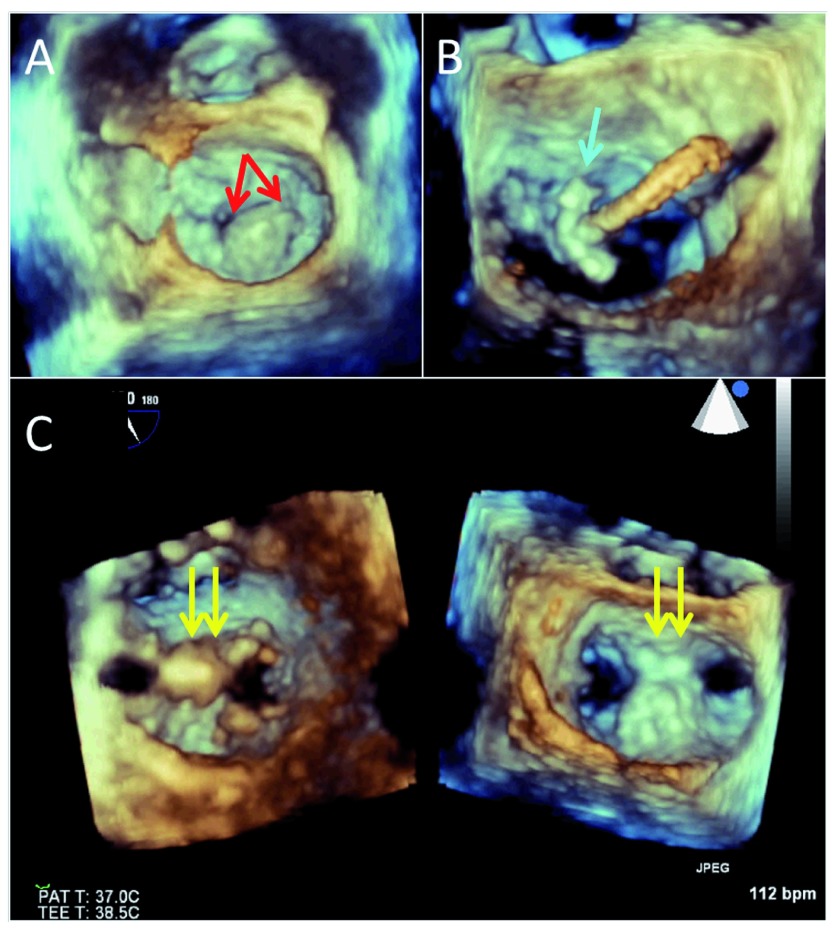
Three-dimensional echocardiography during a transcatheter mitral repair procedure. Panel A shows the baseline mitral valve morphology with a very large prolapsing and partially flail P2 (middle) scallop (red arrows). Panel B shows positioning of the MitraClip device (blue arrow). Panel C is a dual plane three-dimensional image (ventricular and atrial views) of the final, 2-clip (yellow arrows) resulting double orifice. There was trivial residual mitral regurgitation.
